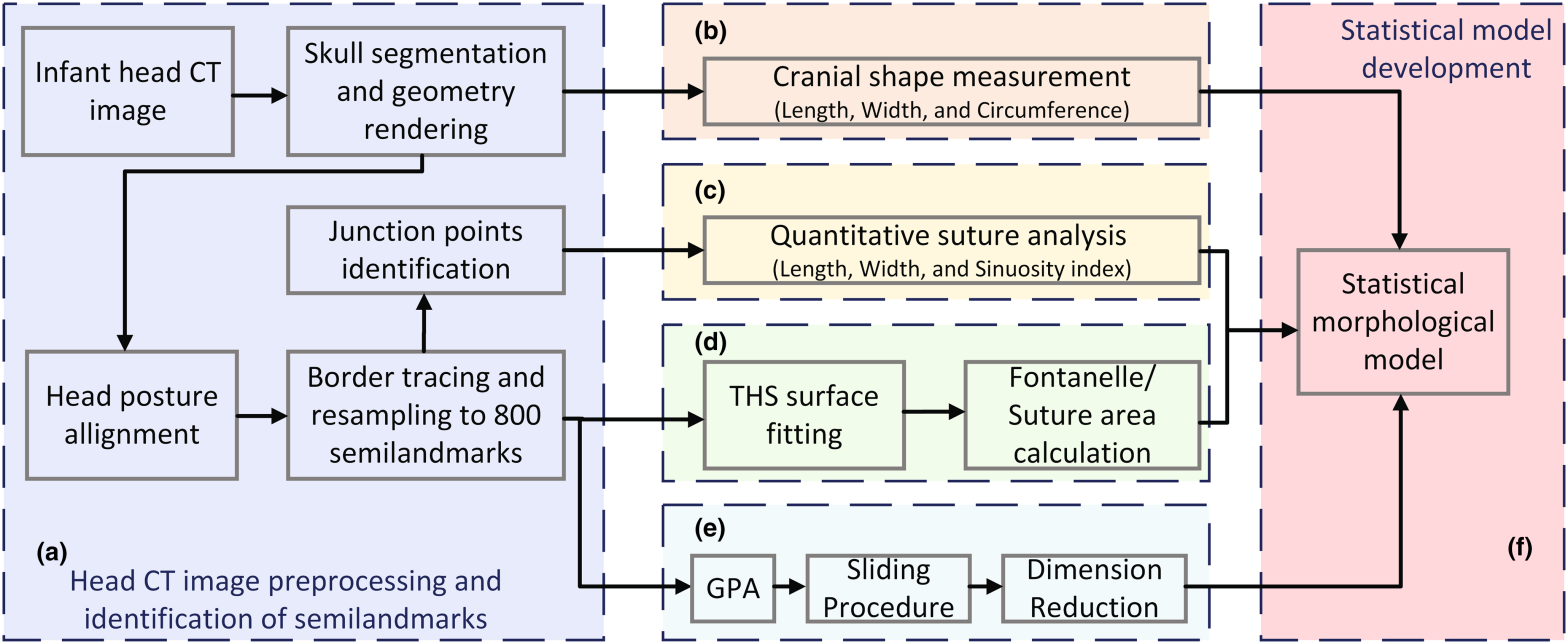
Framework for quantitative morphological analysis of infant cranial sutures and fontanelles, comprising primarily six parts: (a) preprocessing of infant head CT images, (b) cranial shape measurement, (c) measurement of cranial sutures, (d) surface reconstruction, (e) dimension reduction (GPA stands for Generalized Procrustes Analysis), and (f) development of a statistical model.
